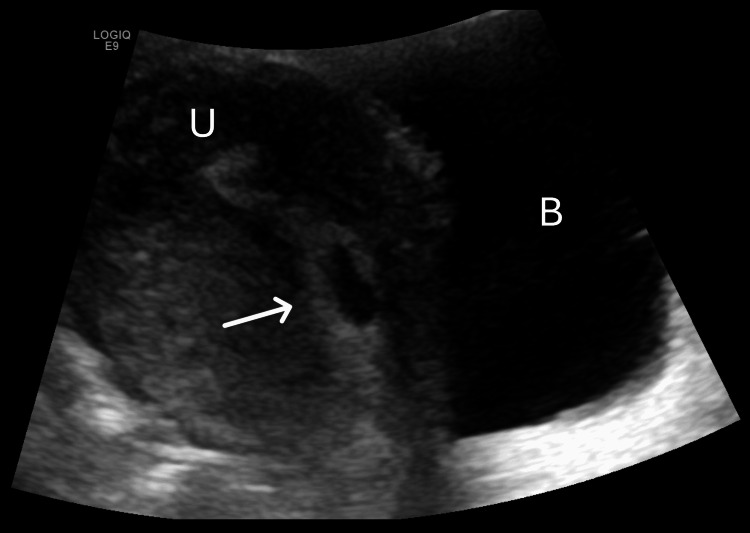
Transvaginal ultrasound, sagittal view Retained products of conception (arrow) were visualized within the lower segment of the uterus. U: uterus; B: bladder
